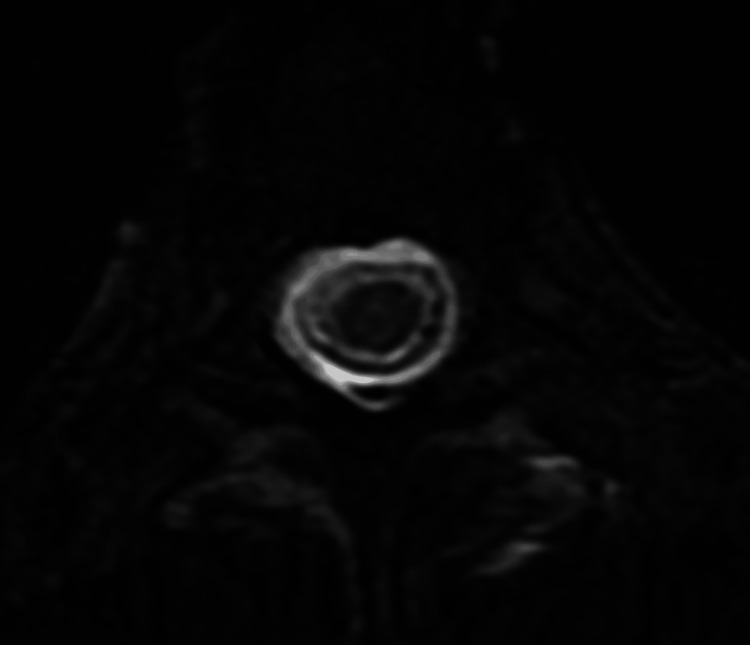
The fat-suppressed T2-weighted axial image at admission. The fat-suppressed T2-weighted axial image shows that the water signal in the epidural space and the dural sac.
